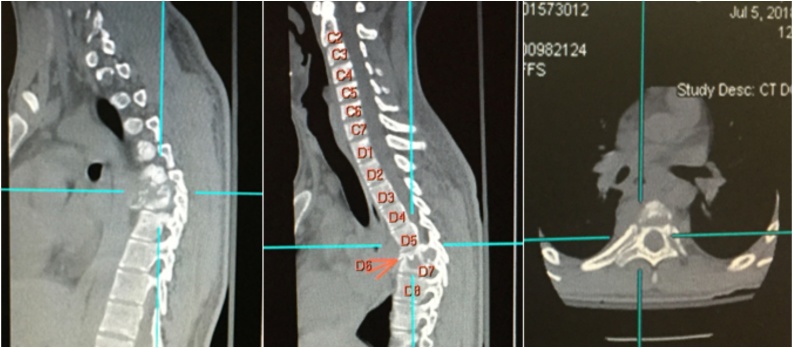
Pre-operative CT scan sagittal and axial images showing complete collapse of D6 and partial collapse of D7 vertebra along with kyphotic deformity.
